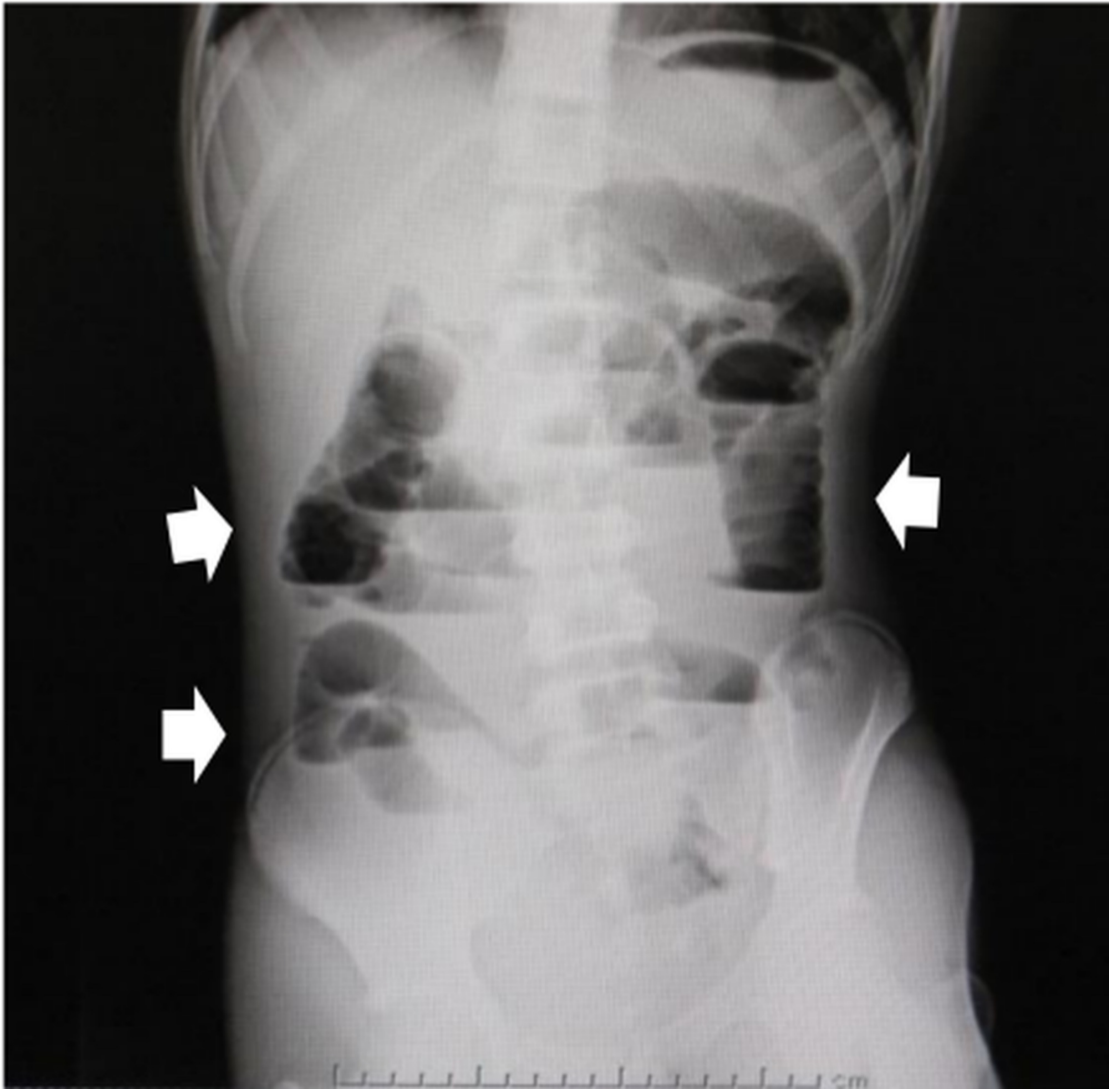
Abdominal X-ray Plain radiography shows dilatation and a stair-step pattern of the small intestine.
